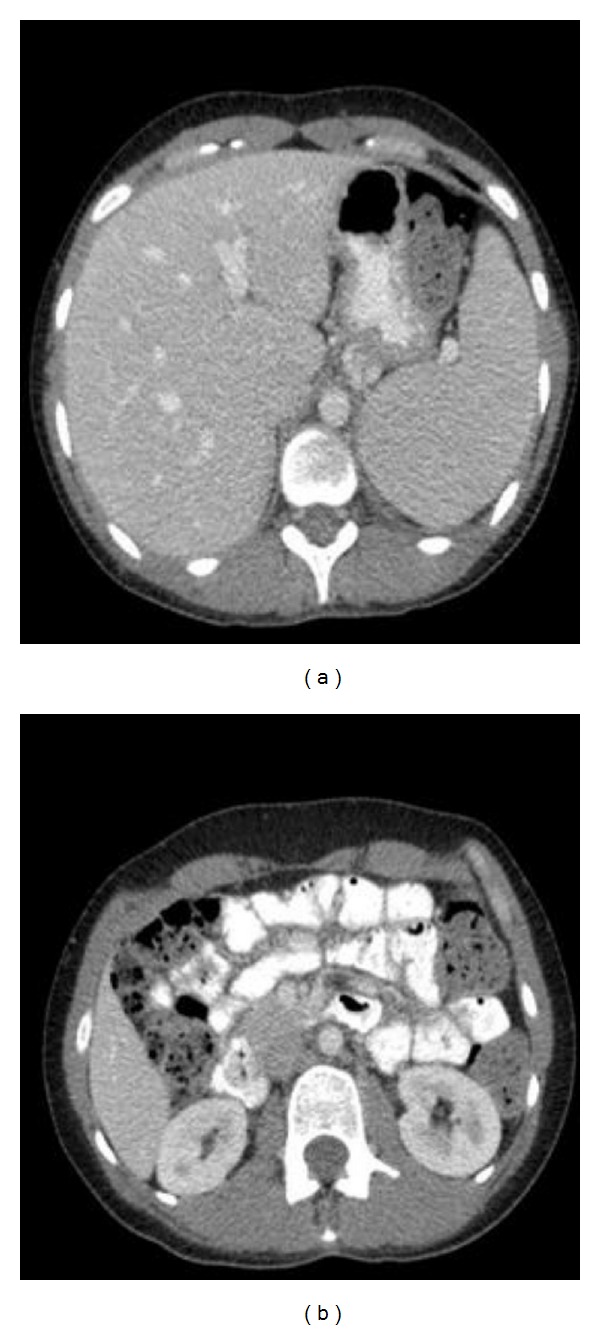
Computed tomography showing resolution of ascites and small bowel thickening three months after discharge from hospital.
